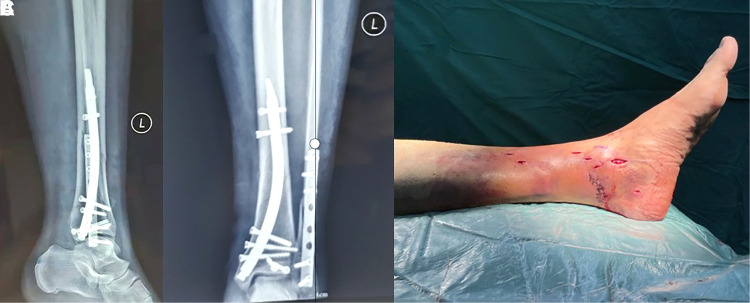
(A) X-ray orthopantomogram at one month after1 closed reduction RTN internal fixation of distal tibial fracture; (B) X-ray lateral radiograph at one month after1 closed reduction RTN internal fixation of distal tibial fracture; (C) surgical incision of the affected limb after closed reduction RTN internal fixation of distal tibial fracture.
